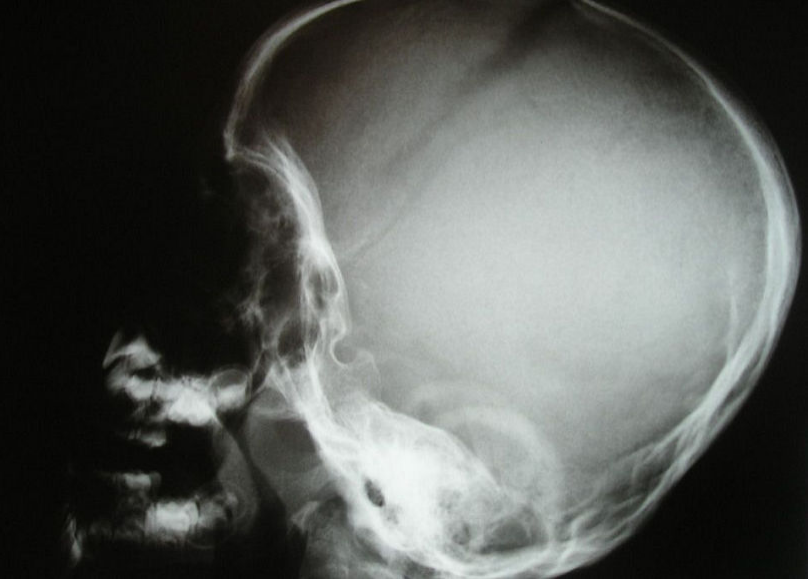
Lateral skull radiograph shows a deformational brachycephalic skull. It is most likely resulted from prolonged lying on the back, however, craniosynostosis was ruled out by clinical and radiographic means. There was thickening and sclerosis of the base of the skull, but a normal density and thickness of the vault was present. The anterior fontanelle is still opened.
